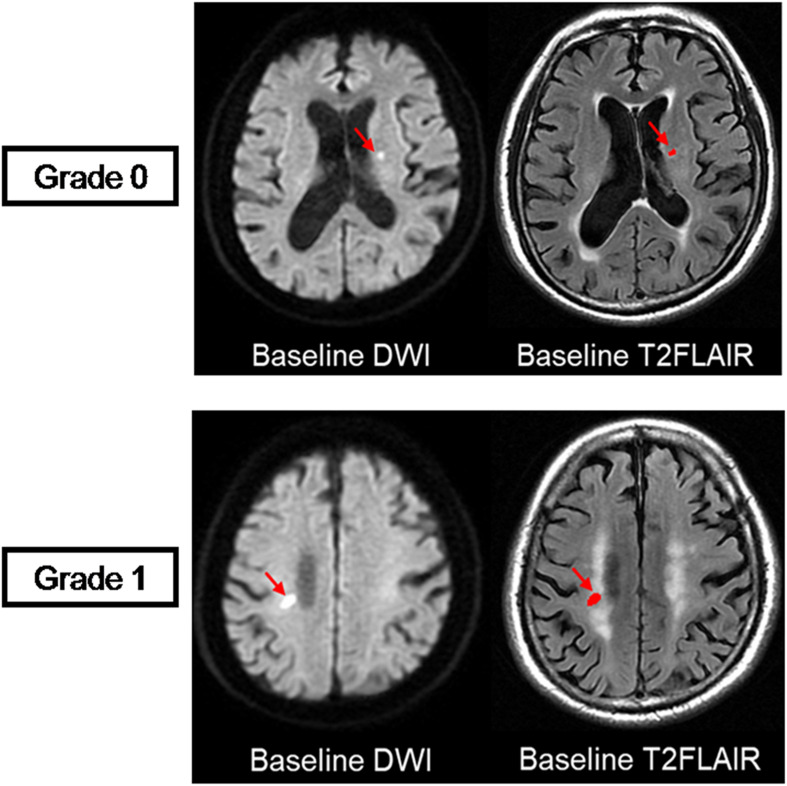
Visual rating scale. Spatial relationship between RSSIs and surrounding white matter hyperintensities are rated at baseline. The top row shows an RSSI on baseline T2 FLAIR (red arrow) isolated from the surrounding WMH, which is rated as grade 0. The bottom row shows an RSSI on baseline T2 FLAIR (red arrow) contacting with the surrounding WMH, which is rated as grade 1.
